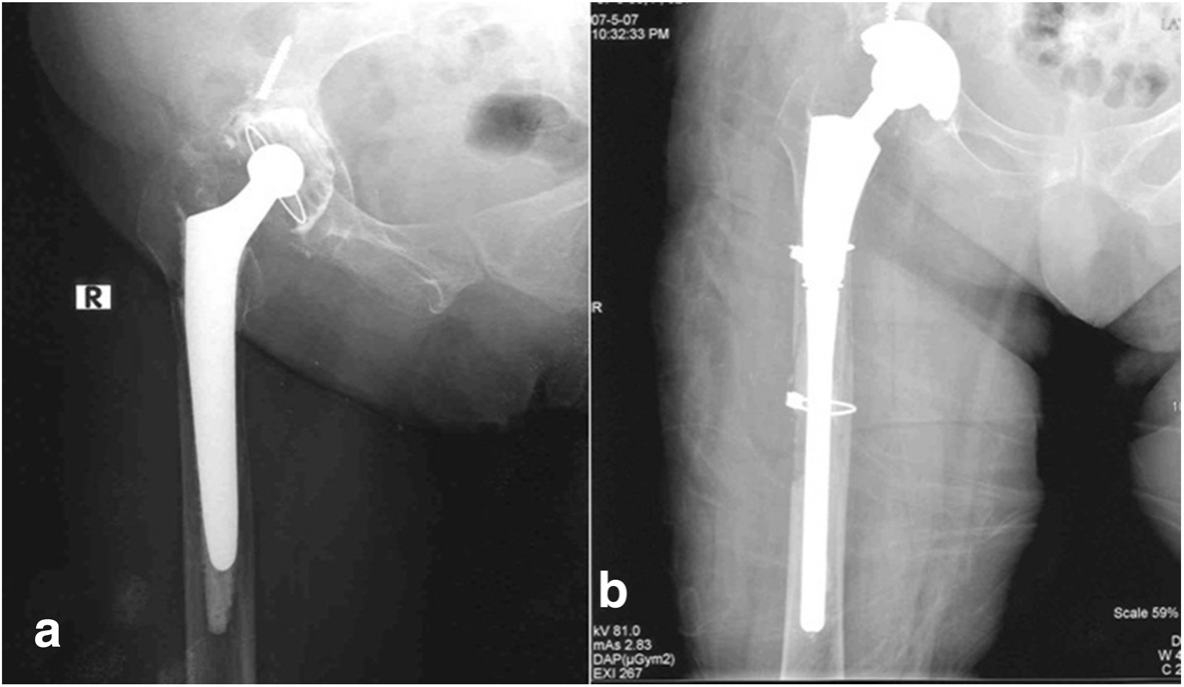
a A 62-year-old female underwent cemented total hip arthroplasty for coxarthrosis secondary to a previous operation for an acetabular fracture. Radiograph shows loosening of the femoral stem and acetabular cup. b Postoperative radiograph of the revised components. The trochanteric osteotomy flap was sufficiently long to allow good access to the medullary canal to remove the cement and to press-fit implant the stem perfectly
